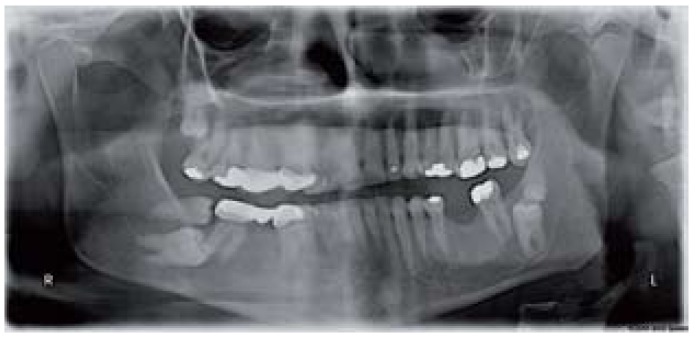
Radiographic appearance of multiple distomolar. An unusual case of kissing molars was also showed.
